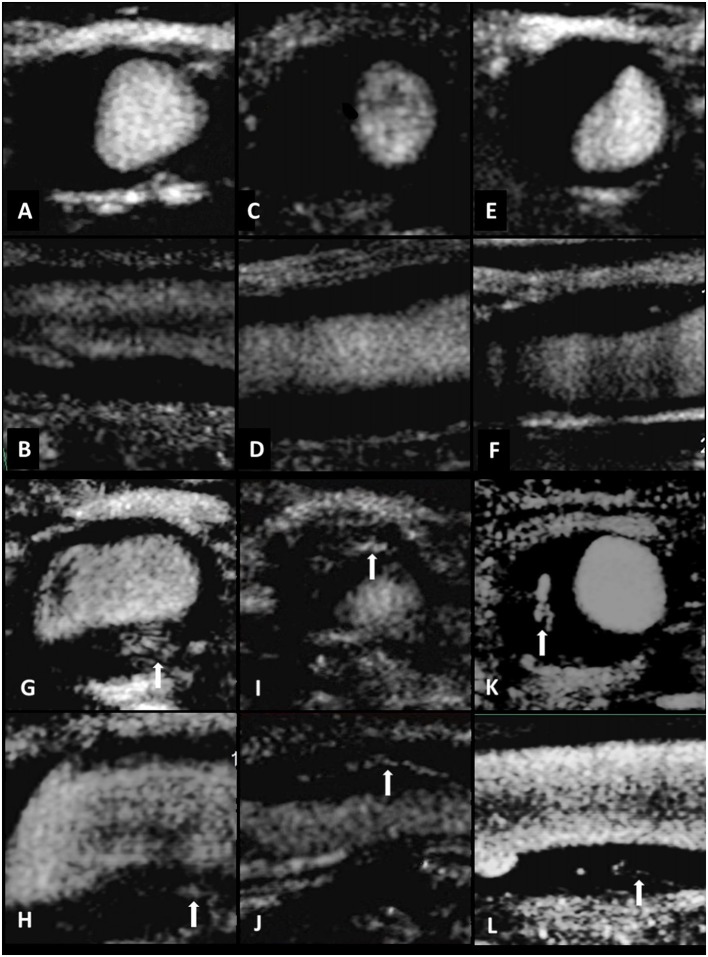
Standard carotid artery plaque ultrasound and SMI in three individuals. Transverse and longitudinal carotid SMI ultrasound of the CCA (A–F). Limited neovascularization was observed within the plaque, suggesting intraplaque vascularization of SMI Grade 1 (A–F). Standard carotid artery plaque ultrasound and SMI in three individuals. Transverse and longitudinal carotid SMI ultrasound of the CCA (G–L). Multiple short-line or strip-like hyperintense echo could be observed both in transverse and longitudinal direction. The arrows indicate moderate or marked neovascularization within the plaque, indicating intraplaque vascularization of SMI Grade 2 (G–L).
